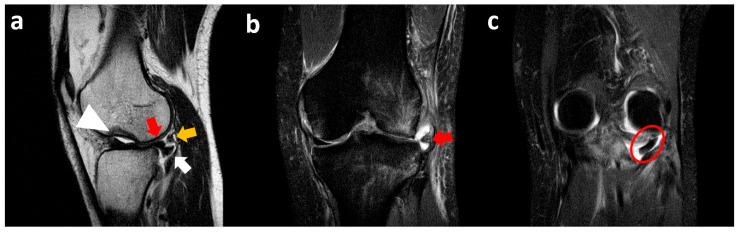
Left knee sagittal T2-weighted MR image (a) and coronal fat-suppressed PD MR image (b) show part of the lateral meniscus body (white arrow) dislocated in the popliteal hiatus below the popliteus tendon (yellow arrow) and the rest of the meniscus (red arrow). Coronal fat-suppressed PD MR image (c) shows lateral meniscal body extrusion (red arrow) and the inferior displacement of the meniscal portion (red circle). Bone marrow edema of the lateral tibial plateau and corresponding femoral condyle, together with an articular cortical depression (‘notch sign’—arrowhead in (a)), is also noted.
